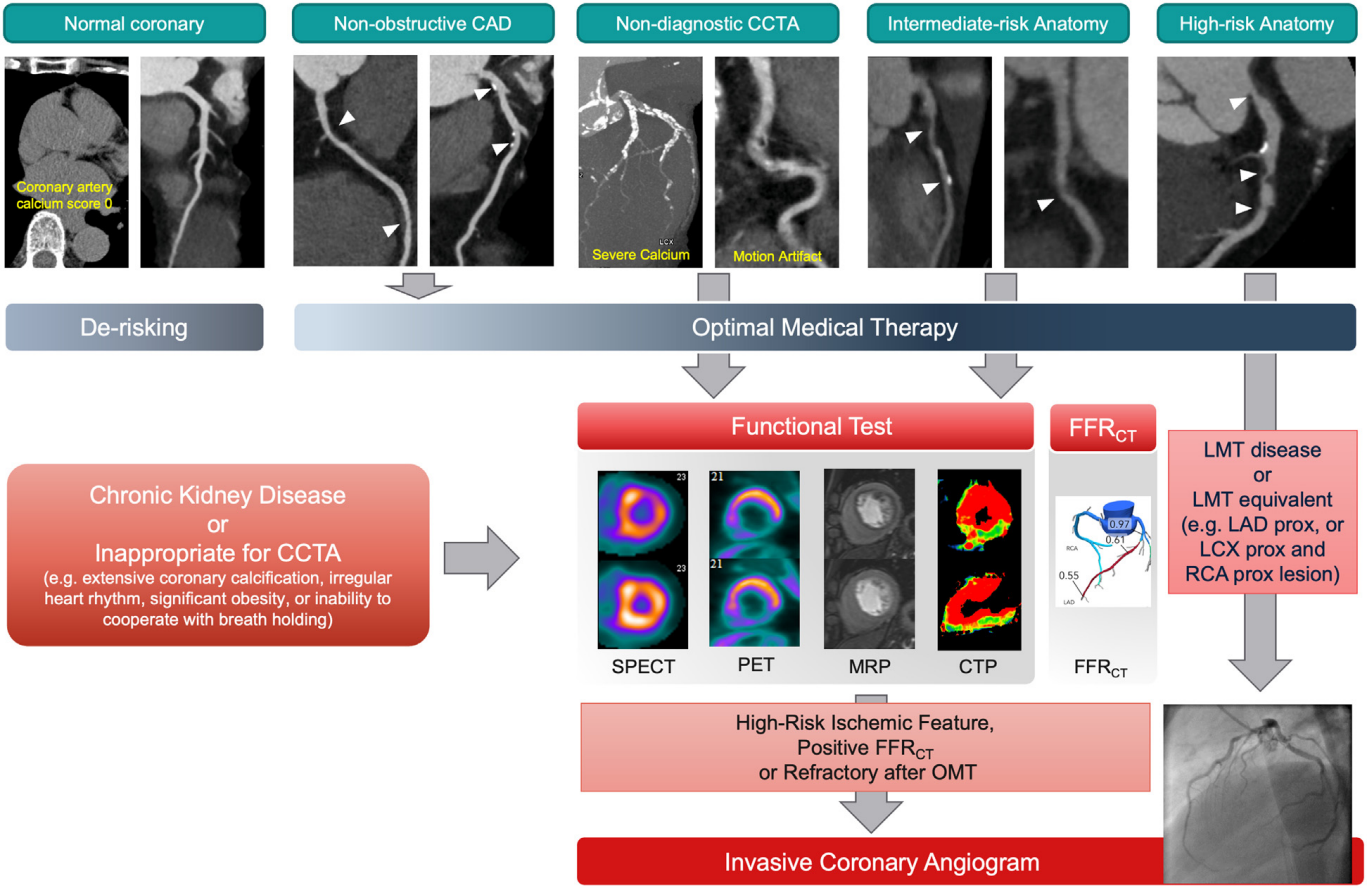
Risk Stratification Using Coronary CTA Patients with zero calcium or normal coronary arteries do not require further testing. Risk factor modification is recommended for all patients with atherosclerotic disease. If coronary computed tomographic angiography (CTA) demonstrates obstructive disease or is nondiagnostic because of severe calcification, further functional testing is recommended. Invasive coronary angiography is necessary when coronary CTA demonstrates left main trunk (LMT) or LMT-equivalent disease. Functional testing can be considered for patients with chronic kidney disease, uncontrollable arrhythmia, heart rate, significant obesity, or inability to cooperate with deep breath-hold. CTP = computed tomographic perfusion; FFR_CT_ = computed tomographic angiography–derived fractional flow reserve; LAD = left anterior descending coronary artery; LCX = left circumflex coronary artery; MRP = magnetic resonance perfusion; OMT = optimal medical therapy; PET = positron emission tomography; prox = proximal; RCA = right coronary artery; SPECT = single-photon emission computed tomography.
